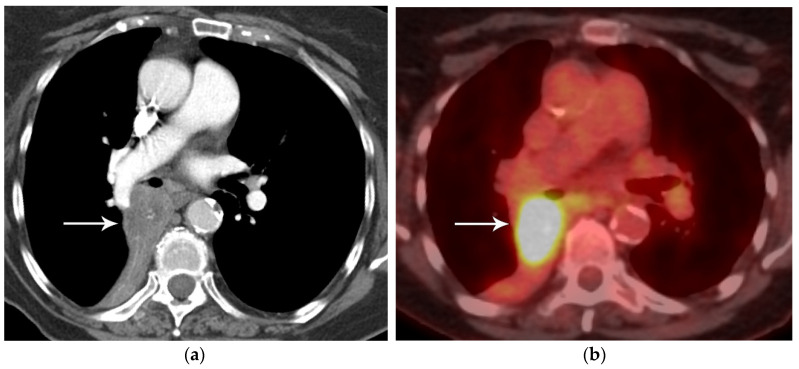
T2 disease. (a) Contrast-enhanced CT shows right lower lobe collapse due to central obstructing tumor (arrow). (b) Axial PET/CT shows FDG avidity of the primary tumor, differentiating it from adjacent atelectatic lung. Tumor size of 3.5 cm is T2a; lobar/lung atelectasis is also T2 disease. As there is no nodal and no distant metastasis, the final stage is stage IB.
